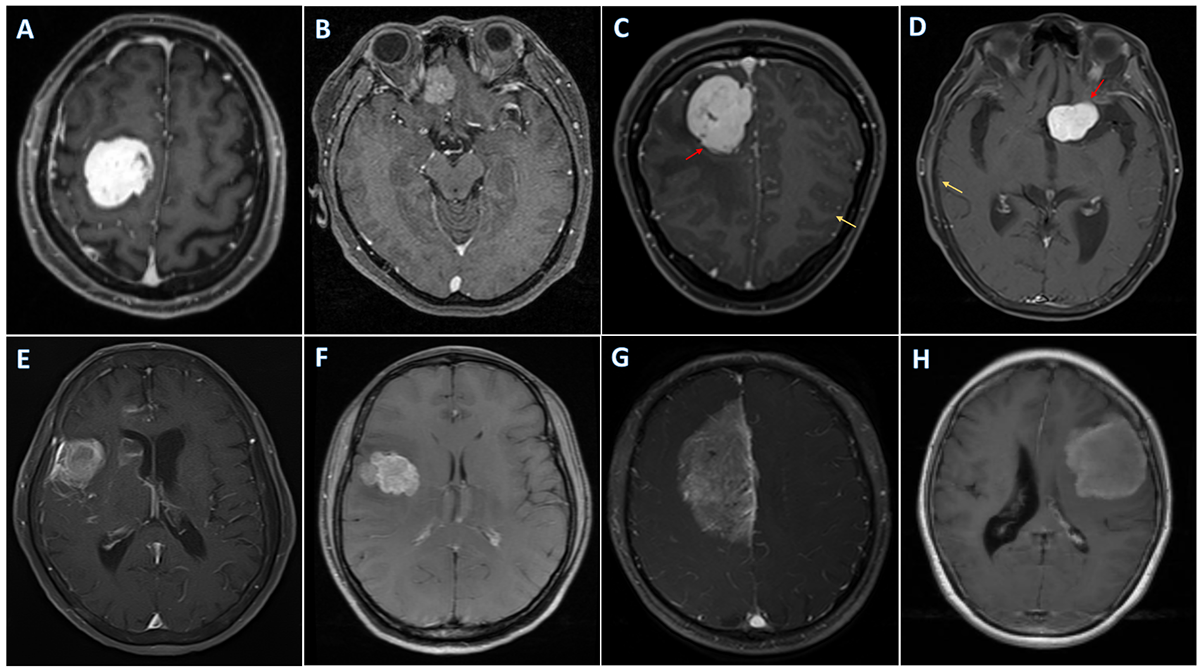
The examples of magnetic resonance imaging from four databases. (A) Center A (magnetization-prepared rapid gradient echos [MPR-AGEs]); (B-C) center B (MPR-AGEs); (D-G) center C (fat-suppressed fast or turbo spin echos [FSEs/TSEs]); (H) center D (FSEs/TSEs). Tumor boundary is more clear in MPR-AGEs as they present high spatial resolution (red arrow). Moreover, the cerebral cortex is rather obvious in MPR-AGEs but not in FSEs/TSEs, as FSEs/TSEs are fat suppressed (yellow arrow).
